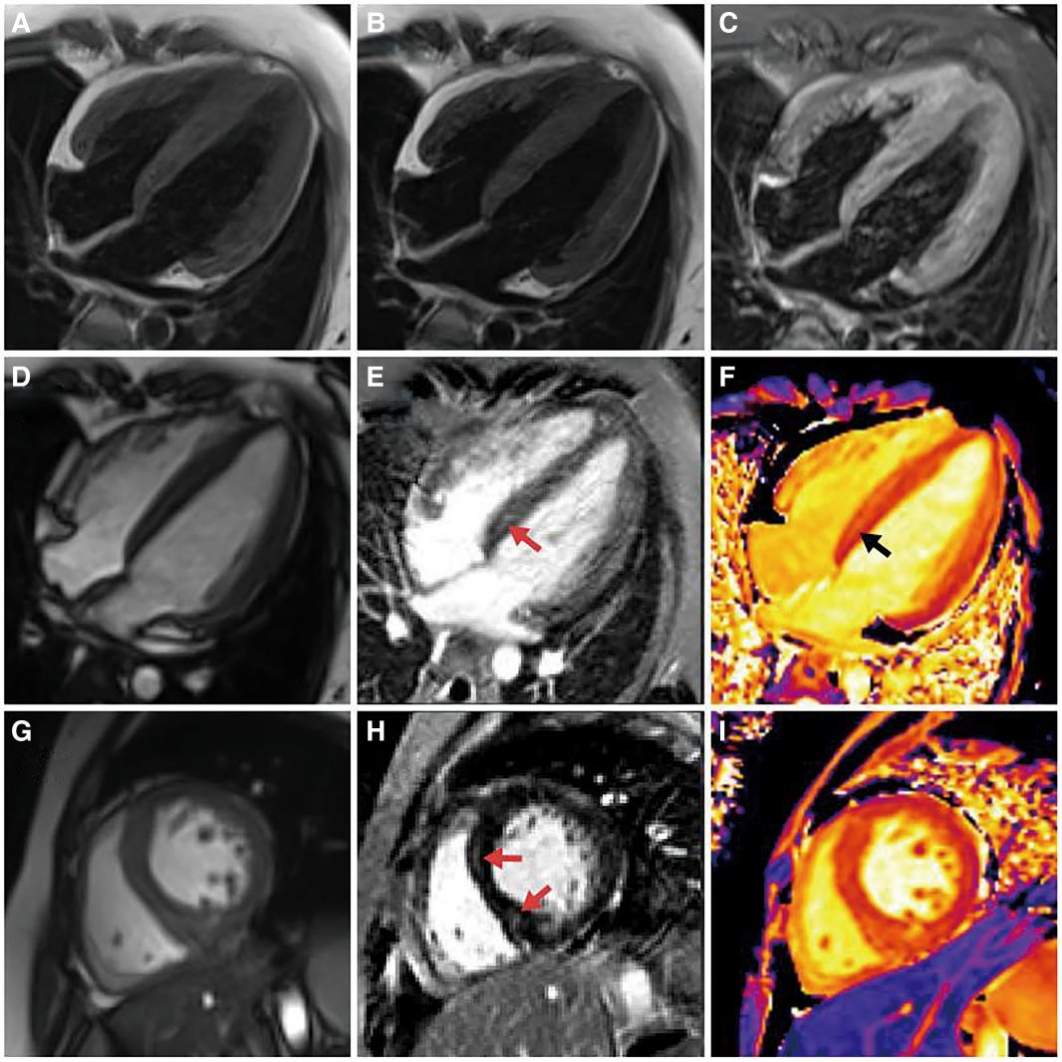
Cardiac magnetic resonance findings with basal septal mid-myocardial late gadolinium enhancement (LGE). (*A–C*) Four chamber black-blood view of T1-weighted image, T2-weighted image, T2-weighted image with fat saturation (T2FS). (*D*) Four-chamber End-diastolic SSFP cine. (*G*) Short-axis end-diastolic SSFP cine. (*E*) Four-chamber LGE image and (*H*) short-axis LGE image demonstrates a linear mid-myocardial hyperenhancement in the basal septum (arrow). (*F*) Four-chamber native T1 mapping and (*I*) short-axis native T1 mapping confirms a mildly elevated T1 value (∼1317 ms) in the basal septum (arrow).
